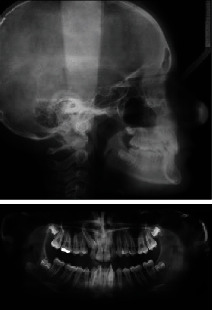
Pretreatment lateral cephalometric and panoramic X-rays.
